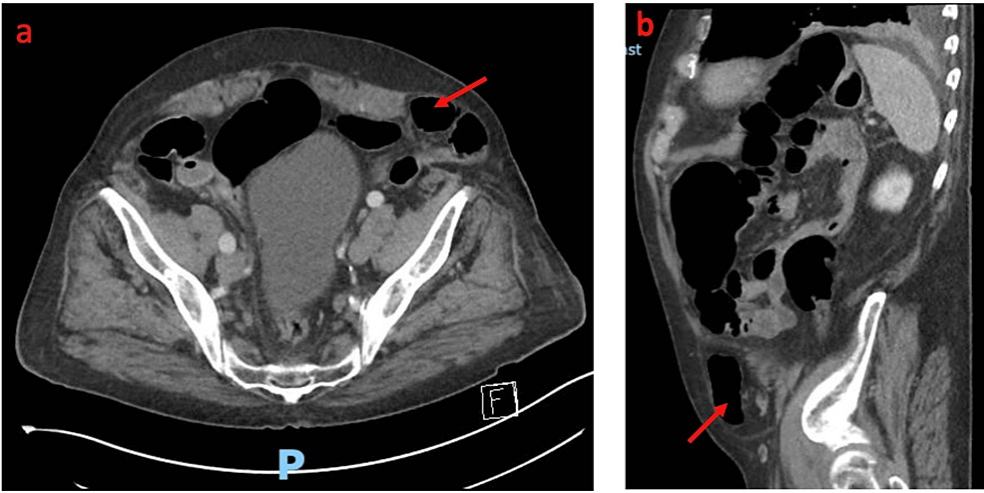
a and b: Axial and sagittal CT abdomen and pelvis with IV contrast showing a sigmoid colon in a left spigelian hernia (red arrows)
